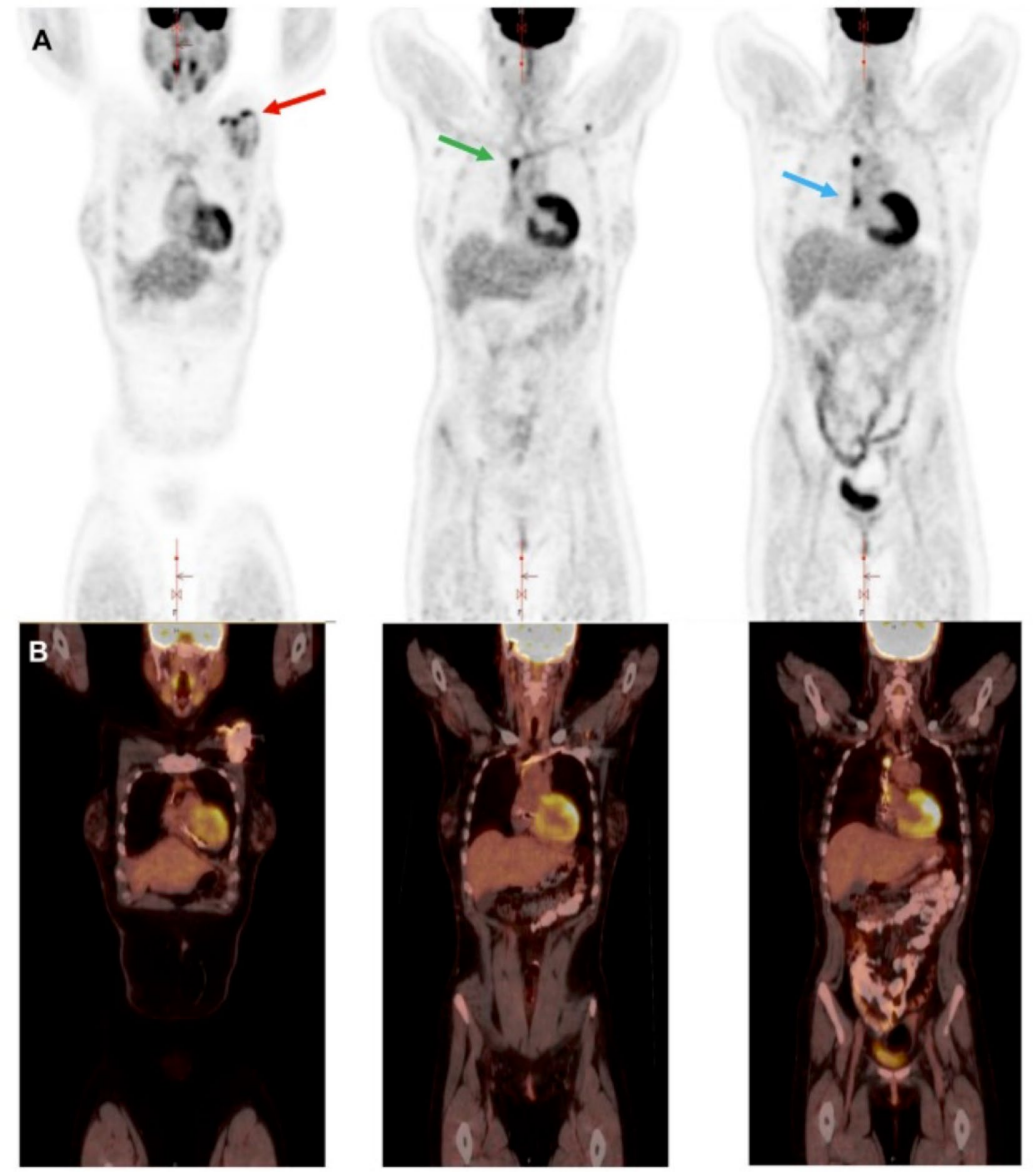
CIED pocket and lead infection diagnosed by ^18^F-FDG PET/CT. Coronal PET images (panel A) show multiple areas of focal, heterogeneous, intense ^18^F-FDG uptake surrounding the ICD generator (standardized uptake value, SUV_max_ 4.8, *red arrow*), along the ICD leads (SUV_max_ 6, *green arrow*) and associated with the ICD leads in the right atrium (SUV_max_ 5.9, *blue arrow*). Uptake persisted on non-attenuation corrected images (not shown). Fusion with CT (panel B) confirm the anatomic localization. These findings confirmed CIED deep pocket infection with lead involvement. (Adapted from Bourque et al. Journal of Nuclear Cardiology. 2024(34):101786, with permission from Elsevier) [6]
